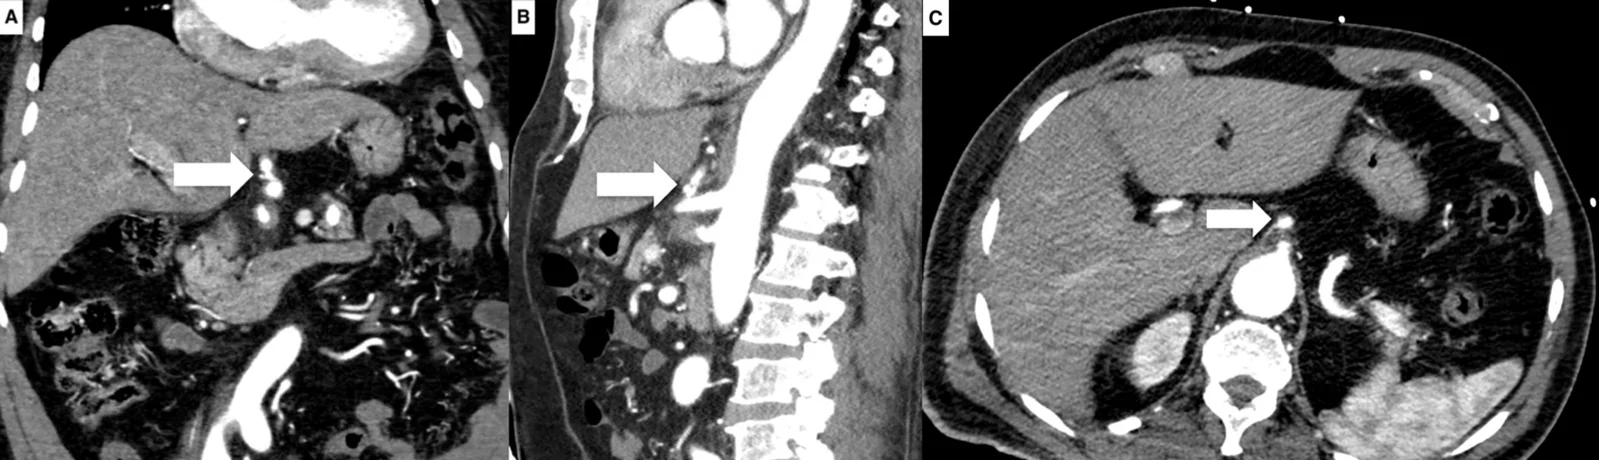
Initial CTA of the abdomen and pelvis obtained on the day of admissions demonstrating a left gastric artery dissection. (A) Coronal, (B) sagittal, and (C) axial views showing the intimal flap and luminal irregularity consistent with dissection CTA: computed tomography angiography
